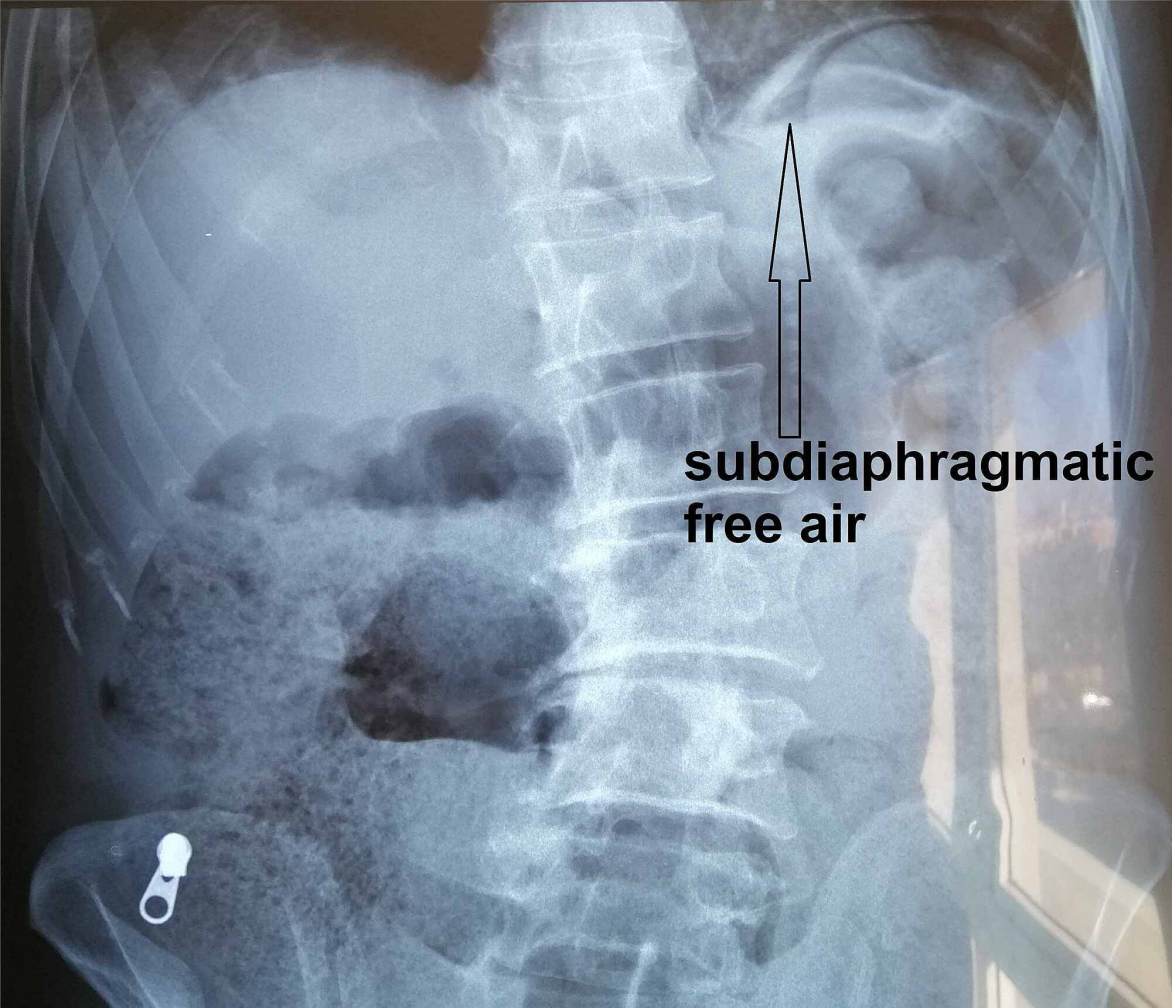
X-ray of abdomen X-ray of abdomen, showing sub-diaphragmatic free air.
